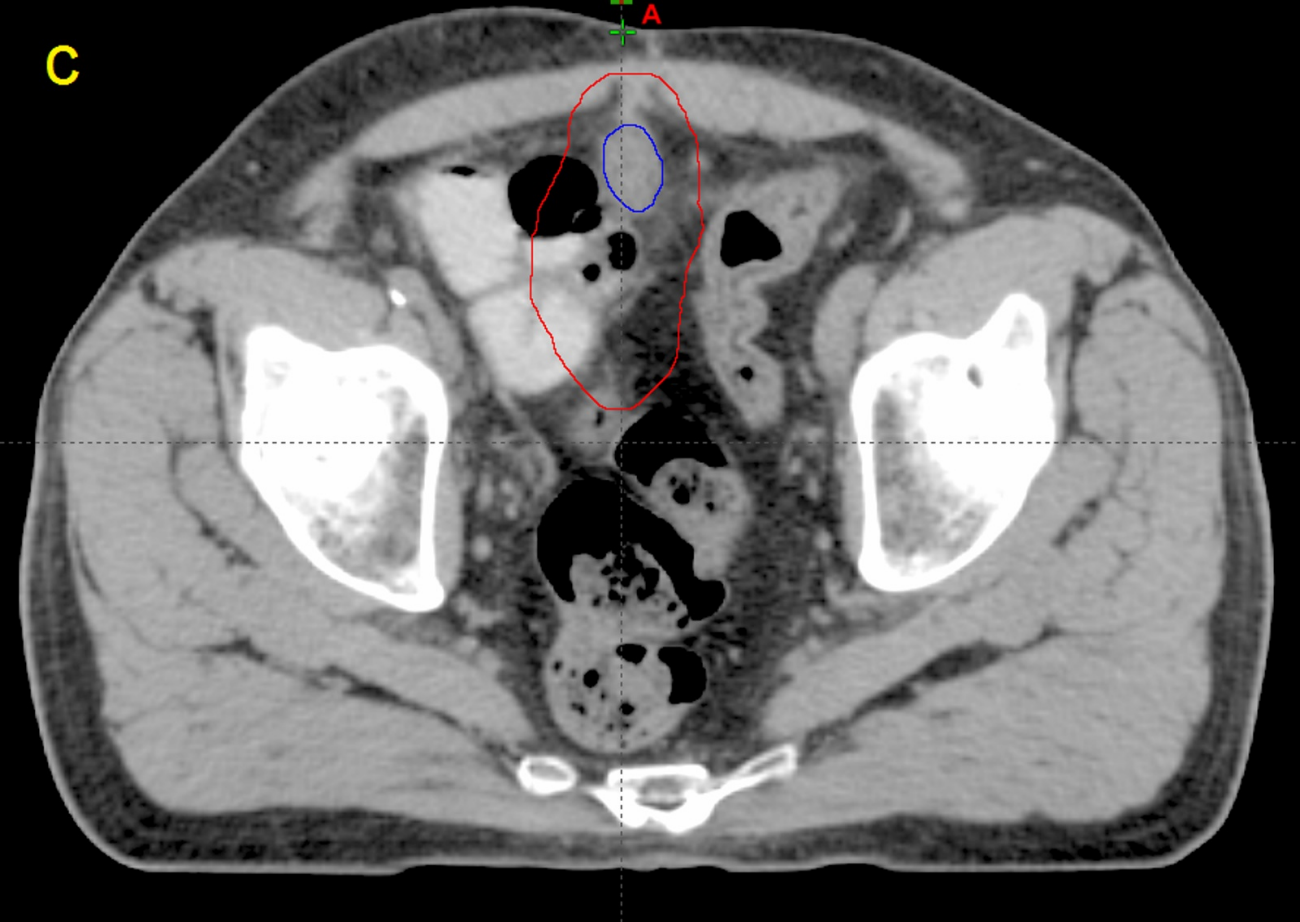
Computed tomographic (CT) image taken from patient P12 c) The planning target volume (red) generated from the contour
